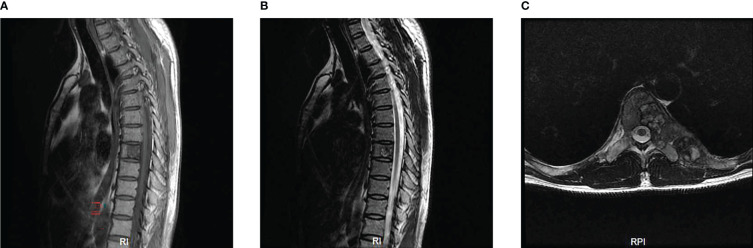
Preoperative MRI scan of the patient’s thoracic spine (A–C). Sagittal scan revealed the density of obvious bone destruction in the T8 on T1 and T2-weighted images (A, B). Transverse MRI scan showed a 6.5cm x 2.0cm sized and well-defined lesion occupying the T8 vertebral body, pedicle and lamina (C).
